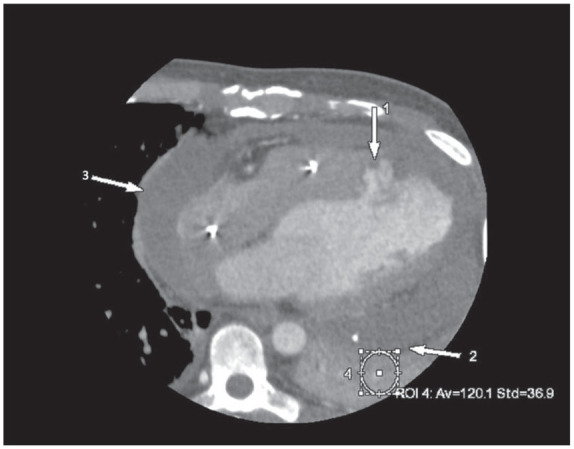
Axial view of coronary computed tomography angiography study with contrast. Arrow 1 indicates the pseudoaneurysm measuring 1.7 cm, which is contained by pericardium at arrow 2 noted at the neck of the apical septum of the left ventricle, consistent with hemopericardium. There is circumferential pericardial effusion at arrow 3. Circle 4 shows an increase in Hounsfield to 120, indicating active extravasation.
